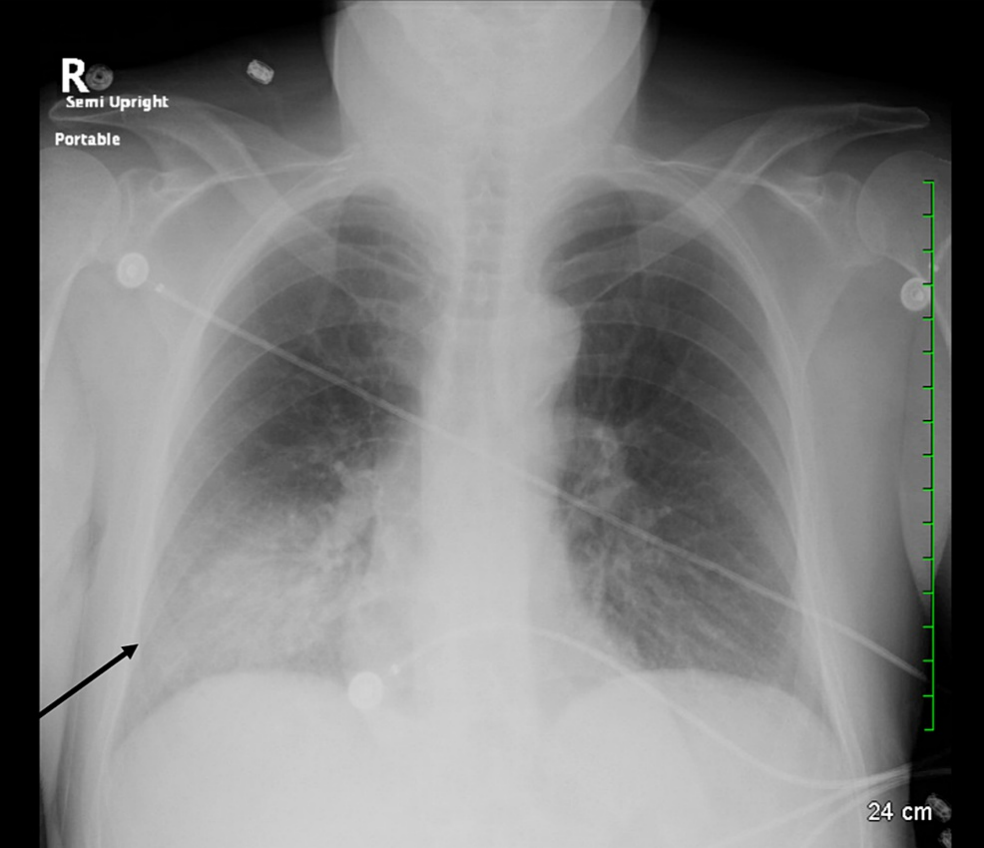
Chest X-ray showing right lower lobe infiltrate consistent with pneumonia.
